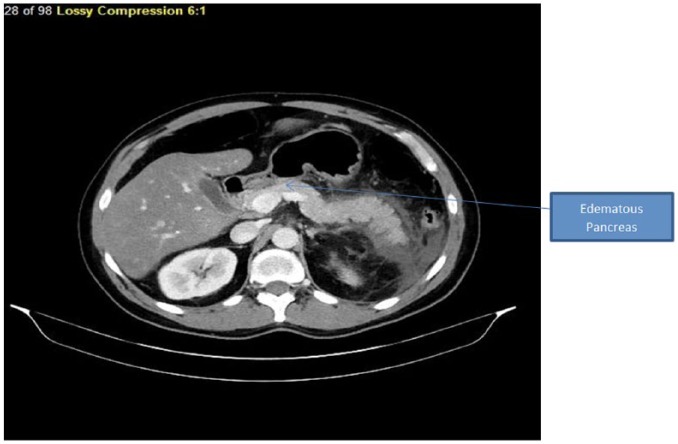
Section of computed tomography scan showing diffuse parenchymal enlargement
